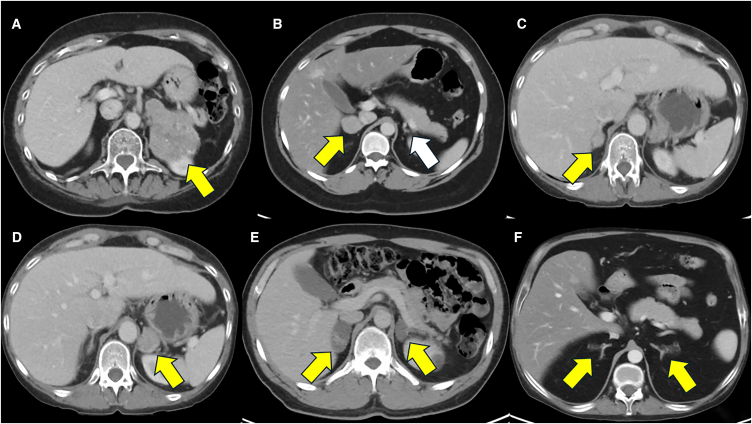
Representative CT scan images from patients with various subtypes of cortisol-producing adrenal lesions causing ACTH-independent Cushing’s syndrome (A) A 70-year-old woman with a large left adrenocortical carcinoma (ACC; yellow arrow), causing severe, overt adrenal Cushing’s syndrome. (B) A 25-year-old woman with a right adrenocortical adenoma (yellow arrow), causing overt adrenal Cushing syndrome (CPA), with a suppressed, atrophic right adrenal gland (white arrow). (C and D) A 43-year-old woman with bilateral CPAs (yellow arrows), causing overt adrenal Cushing syndrome. (E) A 49-year-old man with a bilateral macronodular adrenocortical disease (BMAD) (yellow arrows), causing subclinical Cushing syndrome (mild autonomous cortisol secretion; MACS). (F) A 31-year-old man with a bilateral micronodular adrenocortical disease (BMiNAD) (yellow arrows), causing severe, overt adrenal Cushing syndrome, in the setting of primary pigmented nodular adrenocortical disease (PPNAD). All CT scan images depicted are in venous phase (60 s following contrast injection), according to a modified adrenal protocol of the Carling Adrenal Center and the Hospital for Endocrine Surgery.
